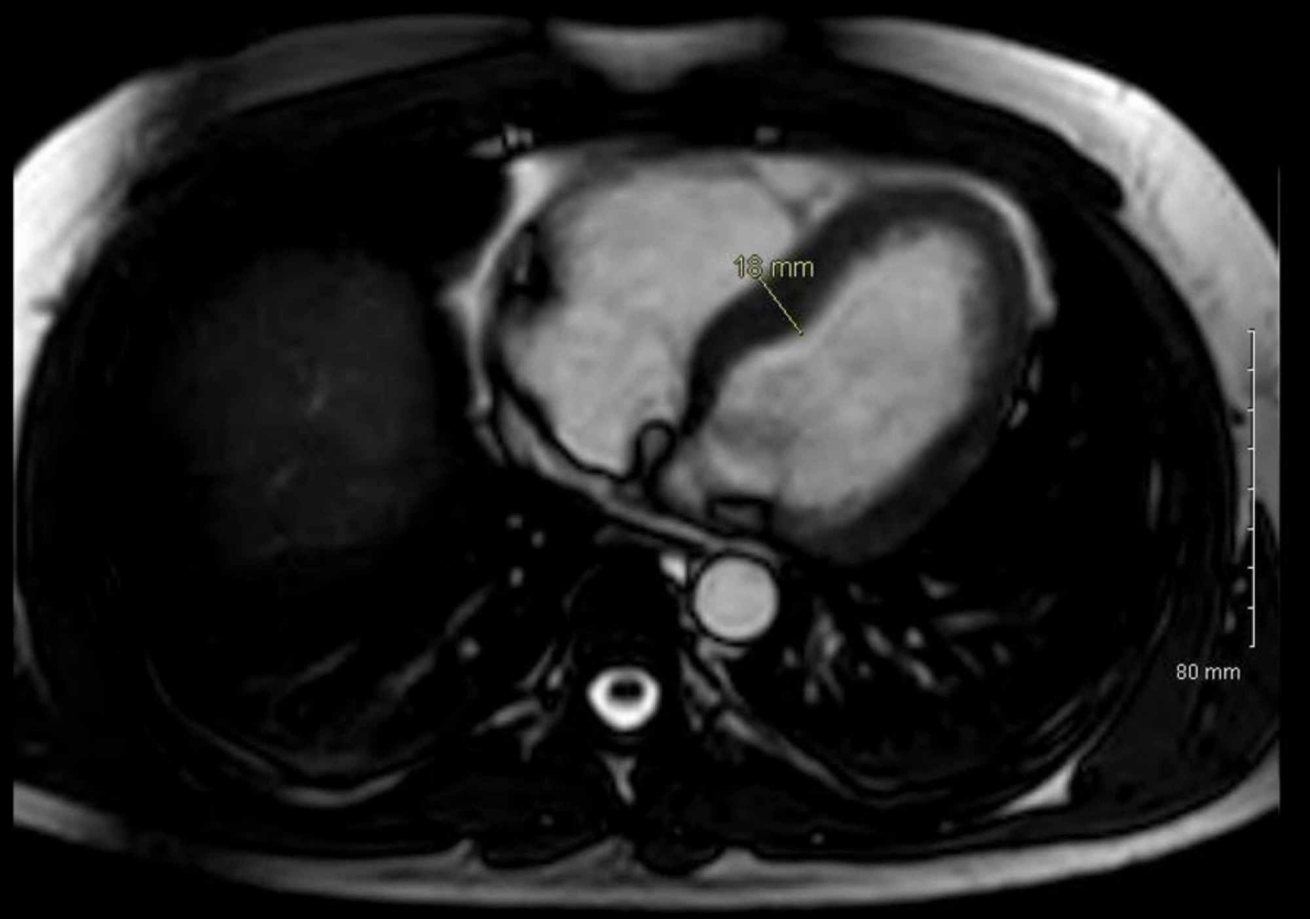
Cardiac MRI demonstrating LVH with septal thickness of 18 mm. LVH, left ventricular hypertrophy.
